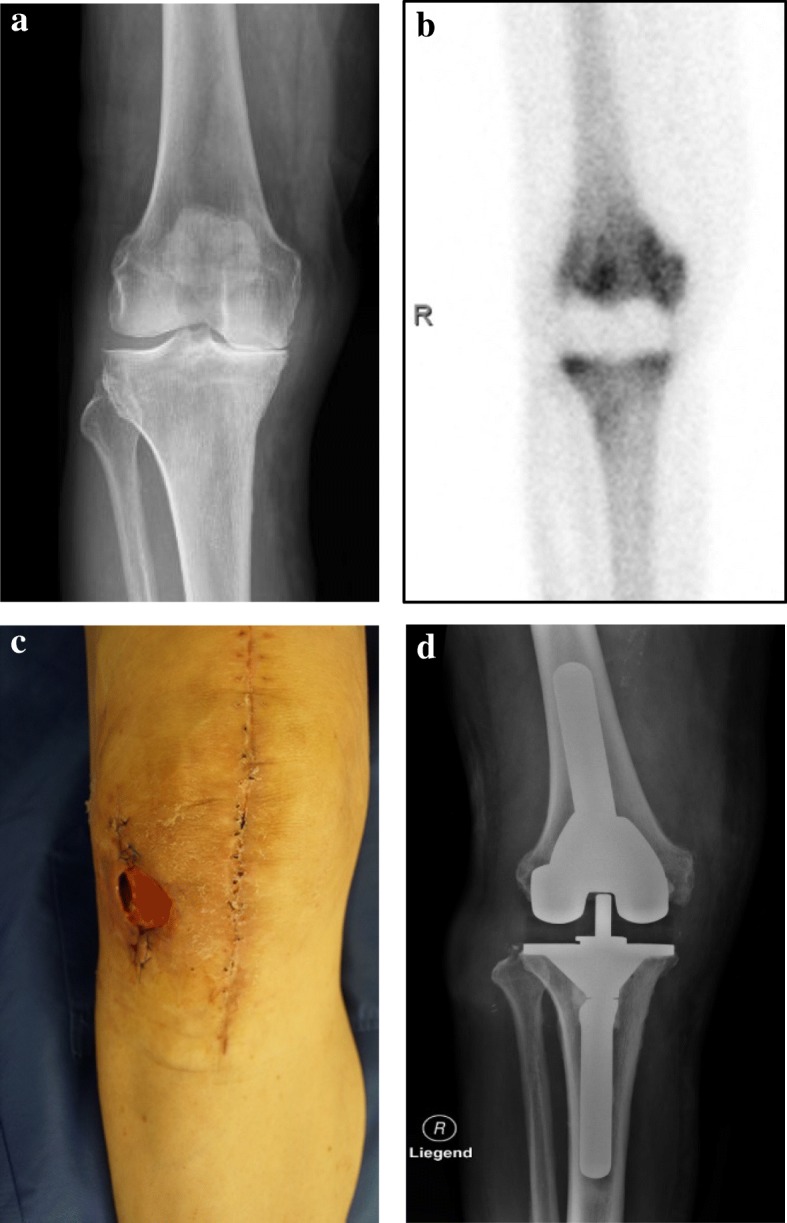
a Preoperative X-ray of the right knee showing osteoarthritis of the knee. b Following the second revision, a bone scintigraphy (Tc^99^m) was performed. This demonstrated increased levels of radiotracer uptake in the distal femur. c The patient developed a wound healing disorder, with a fistula anterolateral to the operational area. d Ten weeks after prosthesis removal, reimplantation involving revision total knee arthroplasty was performed under ongoing antimycobacterial medication
